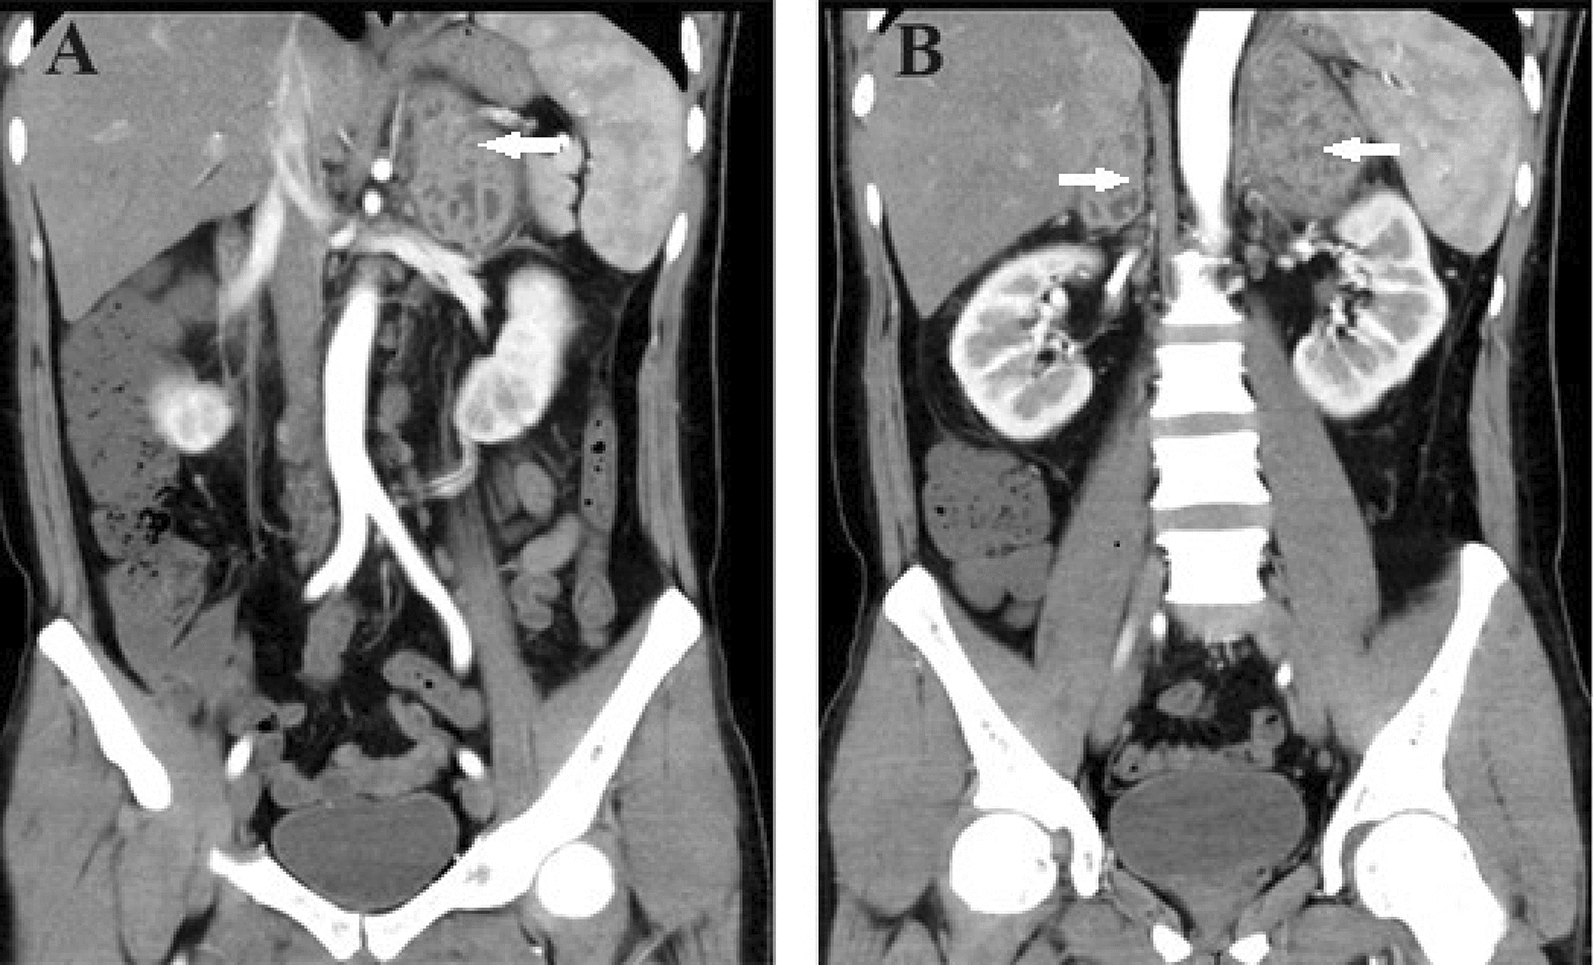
Coronal view of enhanced computed tomography. A Enlargement of lymph nodes around the aorta. B Inability to recognize normal anatomical structures of the adrenal glands because of tumor compression
